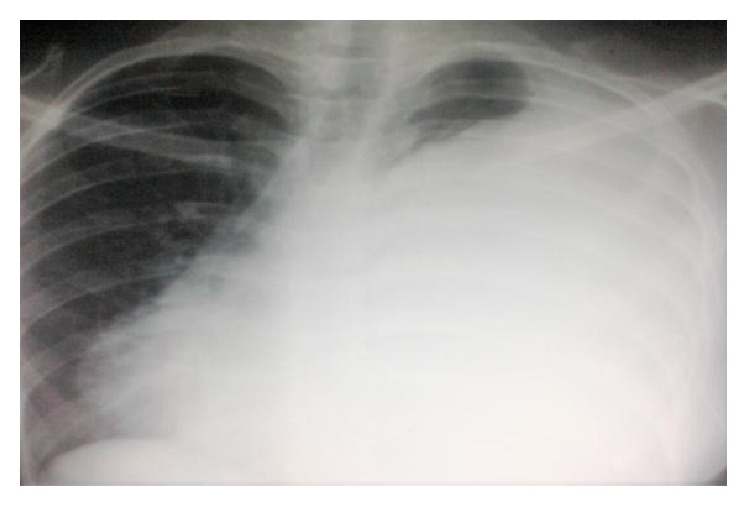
Chest X-ray shows a large opacity of the entire left hemithorax.
